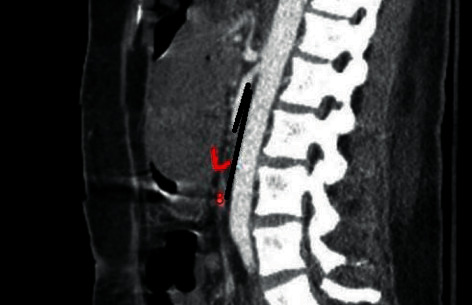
CT sagittal view showing an angle of 8 degrees between the superior mesenteric artery and aorta.
